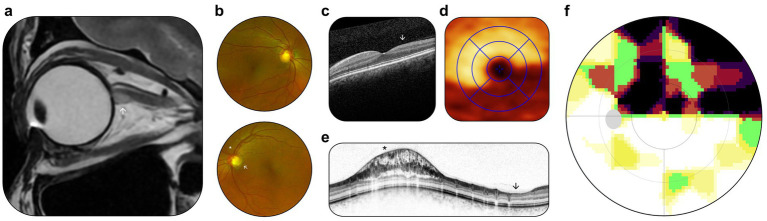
Preoperative outpatient examination. (a) Sagittal T2-weighted MRI scan, lesion (marked by white arrowhead) directly posterior to the optic bulb. (b) Fundus photography, visible optic disk rim notch inferotemporal of the left eye (marked by white arrowhead), and parapapillary retinoschisis (marked by black asterisk). (c) OCT scan, retinal cross-section, reduction in ganglion cell volume (marked by white arrowhead). (d) OCT scan, *en face* view, reduction in ganglion cell volume in inferotemporal sectors. (e) OCT scan, retinal nerve fiber layer, parapapillary retinoschisis (marked by black asterisk), and focal retinal nerve fiber atrophy (marked by black arrowhead). (f) Static perimetry, upper hemifield scotoma, corresponding to ganglion cell layer (GCL) thinning on OCT.
